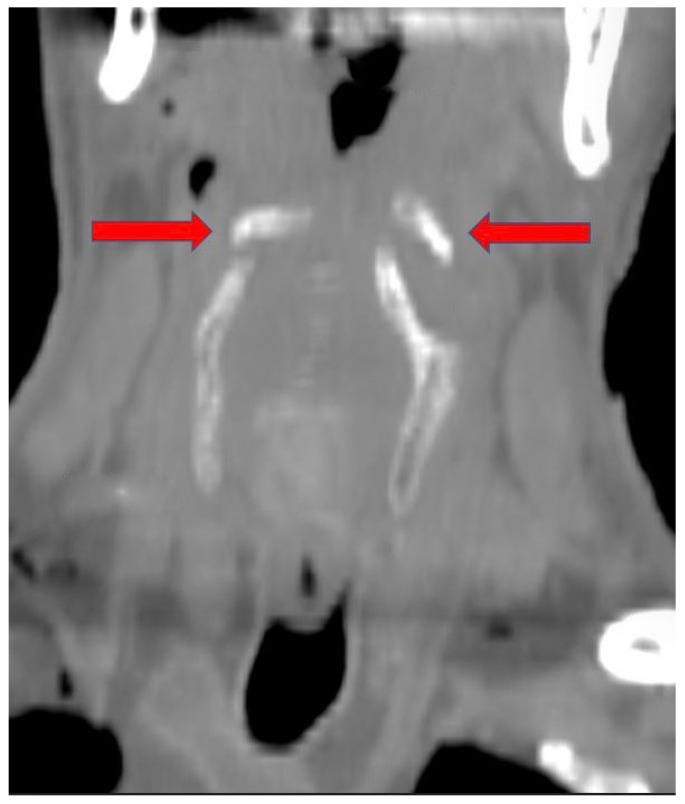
Coronal CT image shows that the dislocation of the two fragments can only be explained by the action of forces acting in the lateral–medial direction (red arrows).
